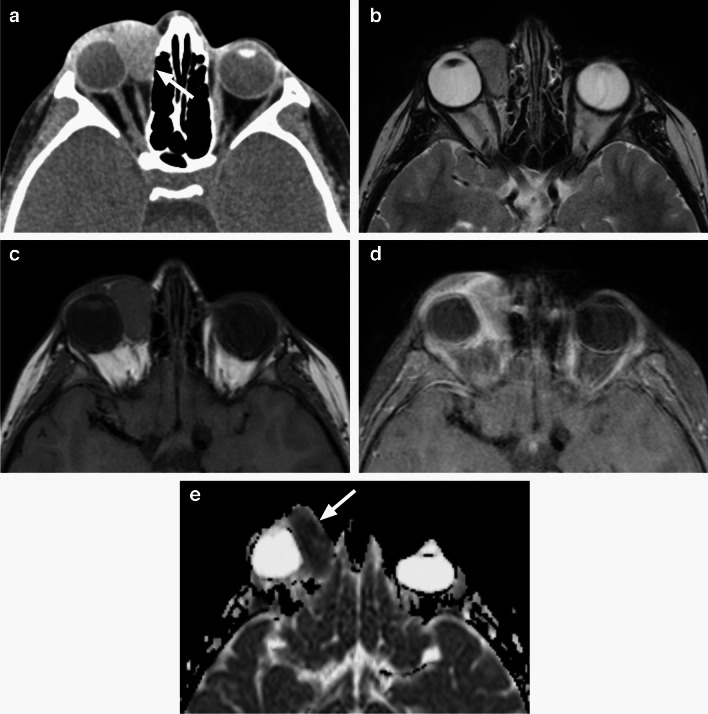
Rhabdomyosarcoma. Axial non-enhanced CT image (a) shows a well-defined homogenous oval extraconal lesion (arrow) in the superonasal aspect of the right orbit, isodense with extra-ocular muscles and mildly compressing the globe. Adjacent medial orbital wall appears intact. Axial T2-weighted (b) and T1-weighted (c) images at a similar level show homogenous intermediate to slightly increased signal intensity of the mass, without flow voids. There is mildly heterogenous enhancement on axial postcontrast T1-weighted image with fat saturation (d, suboptimal image quality due to motion artifacts) and low diffusivity of the mass (arrow), which is hypointense to the brain on corresponding ADC map (e)
